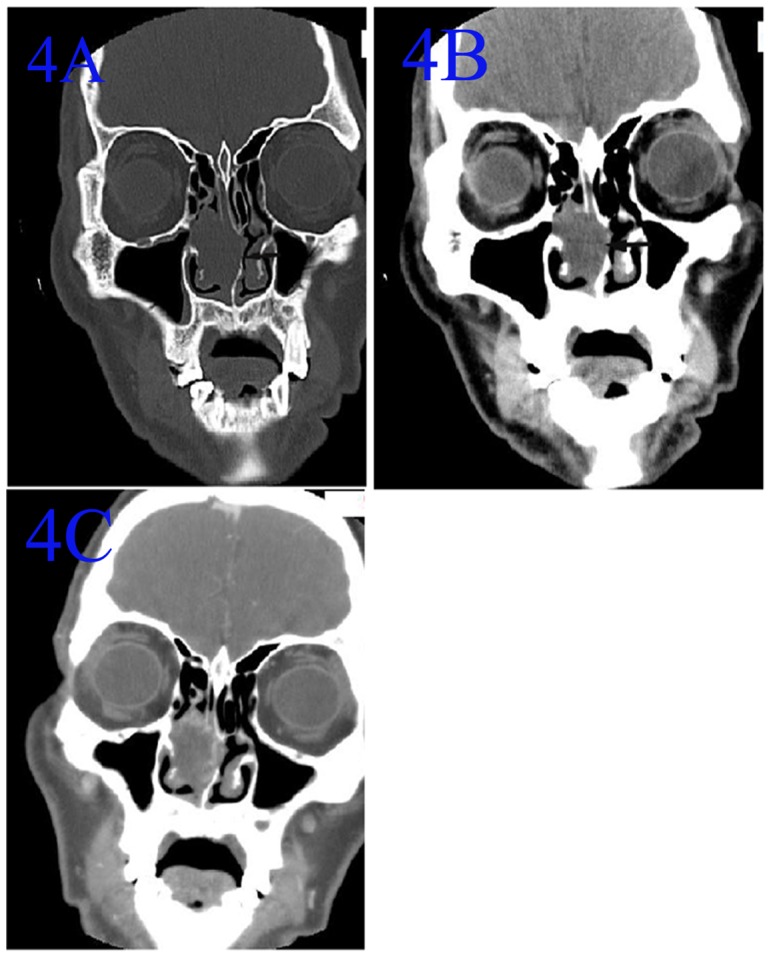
CT of an ANP confined within the right nasal cavity. Noncontrast coronal CT in bone window(A) and in soft-tissue e window(B) showed a soft-tissue mass limited within the right nasal cavity. The edge was irregular, and the nasal septum was compressed and deformed (arrow). The CT value was 26 HU. The walls of the right nasal cavity were intact. The maxillary and ethmoid sinuses were not involved. 4C: Contrast-enhanced CT showed the lesion was non-enhanced.
